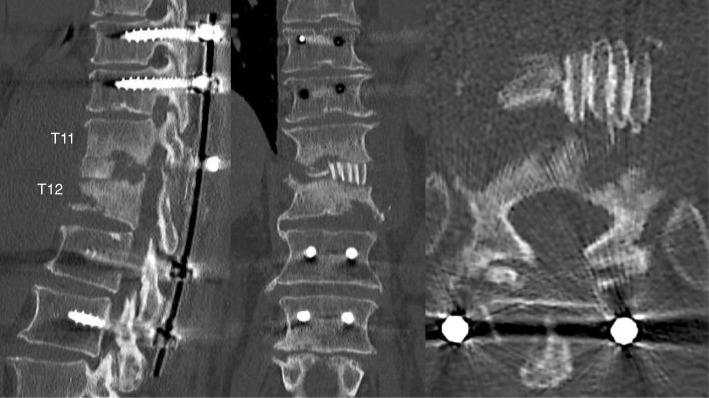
Postoperative computed tomography demonstrated anterior fusion at T11 and T12 with rib bone graft
